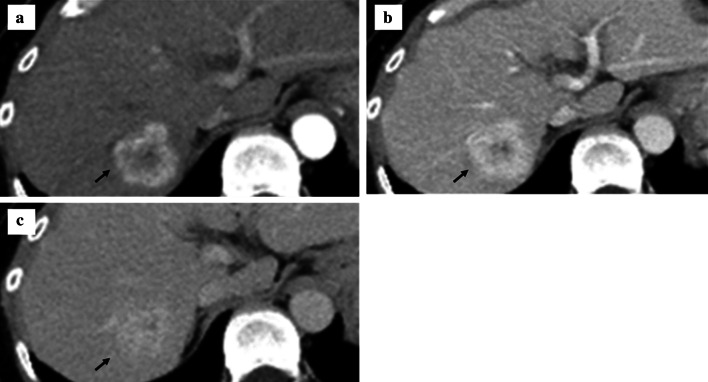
Findings of dynamic abdominal computed tomography. a The arterial phase showing a ring-like enhanced mass at segment 7 measuring 35 mm in diameter shaped like an irregular arrow. b Portal phase. c The enhancement was prolonged to the late phase
